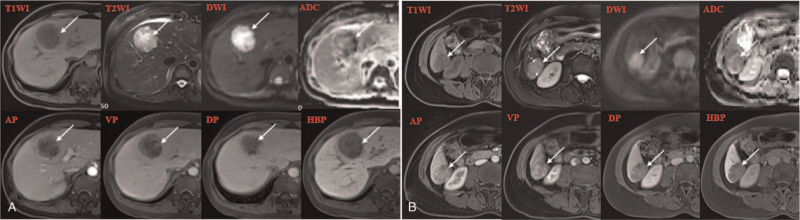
Abdominal magnetic resonance findings of the 2 tumors (white arrows). The tumors in S4 (A) and S6 (B) indicated similar findings of low signal intensity on T1WI and high signal intensity on T2WI. The DWI (b = 800 mm^2^/s) showed high-intensity masses and ADC map from conventional DWI showed the massed with decreased signal intensity, suggesting both tumors diffusion restriction. The S4 tumor showed rim-enhancement throughout arterial, portal venous, and delayed phase. The S6 tumor showed arterial-enhancing and delayed wash-out. The hepatobiliary phase appeared as hypointense nodules. ADC = apparent diffusion coefficient, DWI = diffusion weighted imagings, HBP = hepatobiliary phase, T1WI = T1-weighted images, T2WI = T2-weighted images.
